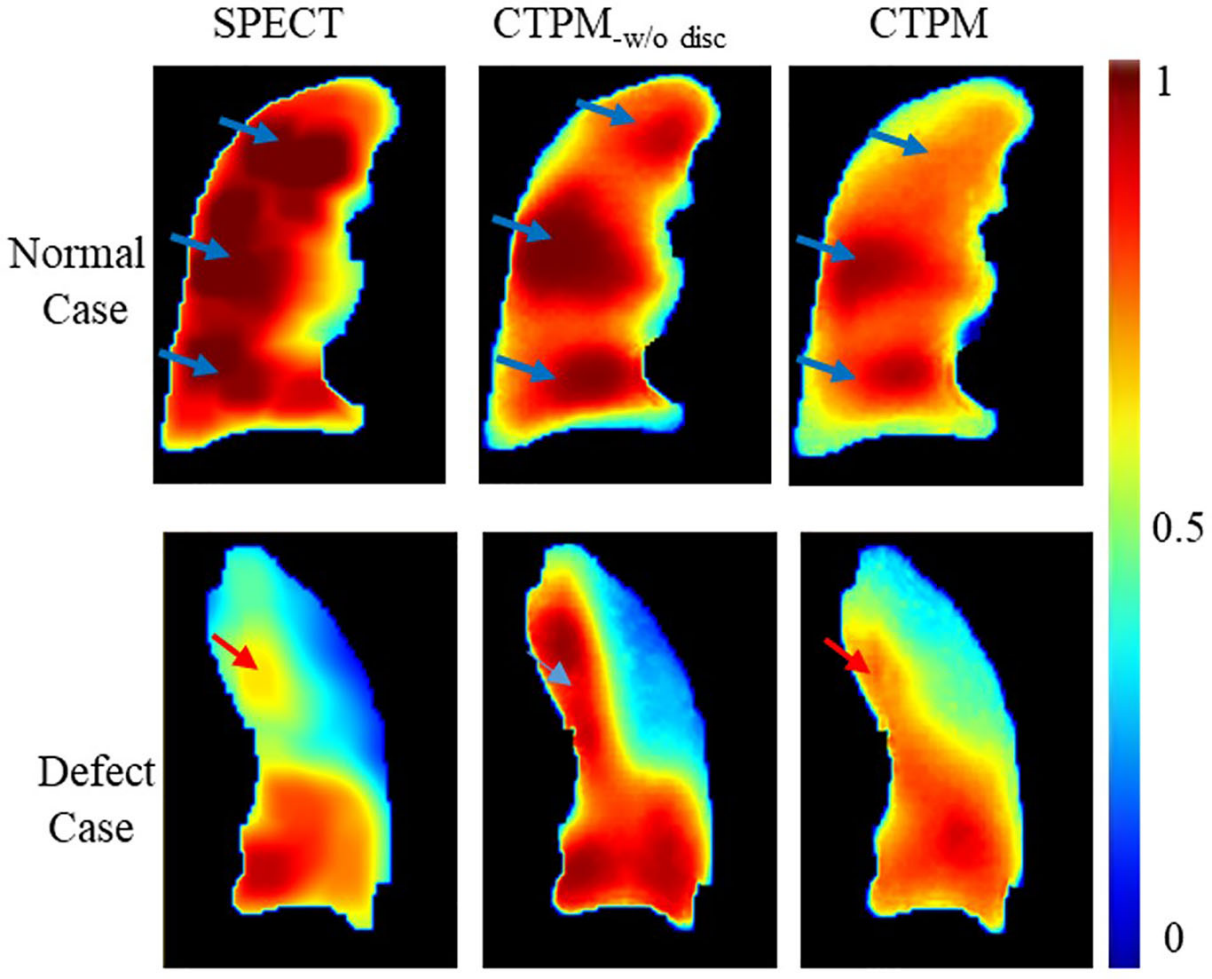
Effects of the SPECT discretization. CTPM_-w/o disc_ indicates prediction without SPECT discretization procedure.
